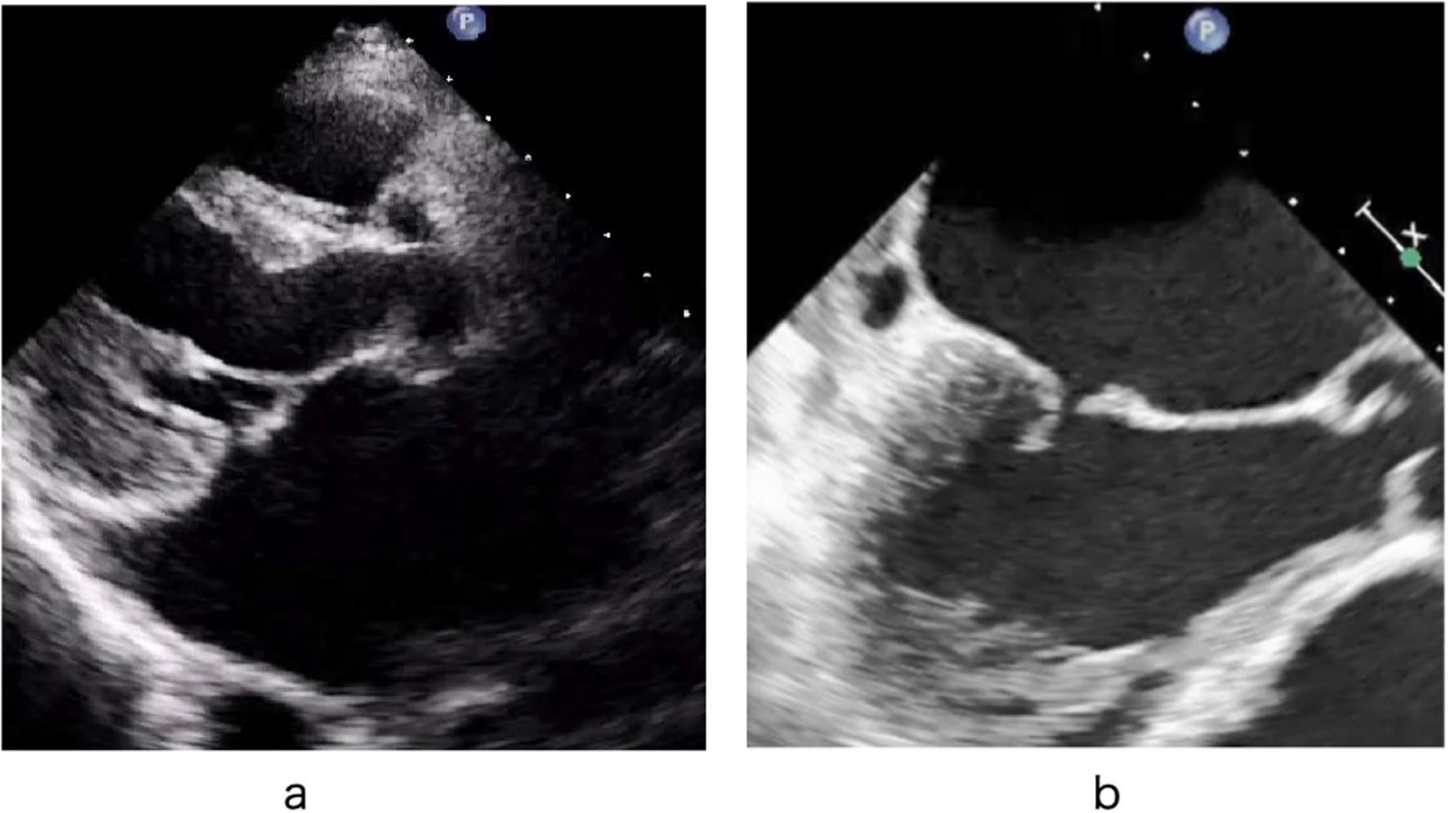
Pseudo-prolapse of the anterior leaflet. a Transthoracic echocardiography. b Transesophageal echocardiography
